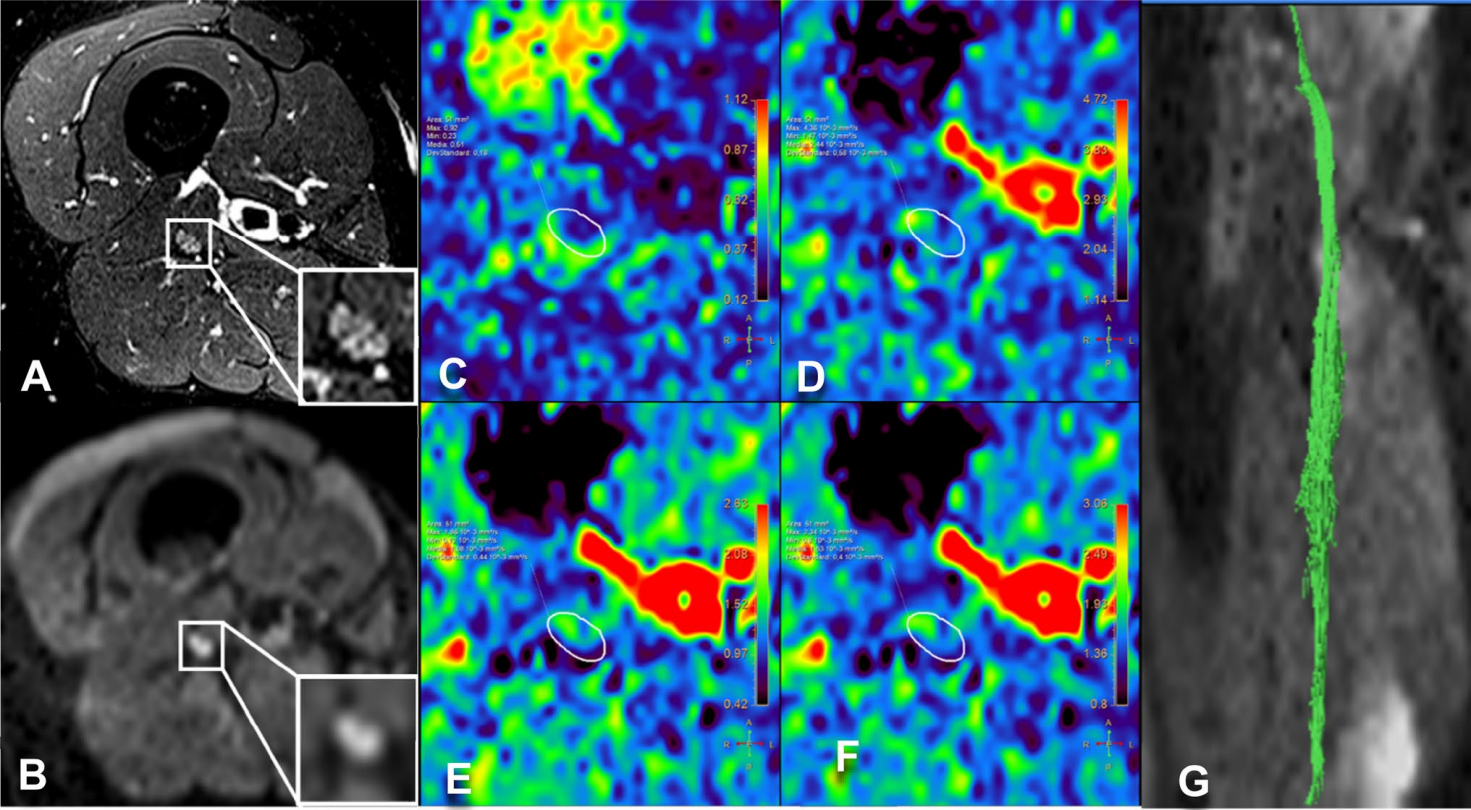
ATTRv-C. MR Neurography, sciatic nerve at mid-thigh. B DWI trace-weighted image (*b* = 700), C FA, D AD, E) RD, F MD maps, G sciatic nerve tractography at thigh. Sciatic nerve CSA = 42.6 mm^2^, NSI = 1.70, FA = 0.51, AD = 2.44 10^–3^ mm^2^/s, RD = 1.08 10^–3^ mm^2^/s, ADC = 1.53 10^–3^ mm^2^/s. Increased size and signal intensity of the fascicles of the sciatic nerve with no significant changes of CSA and FA
